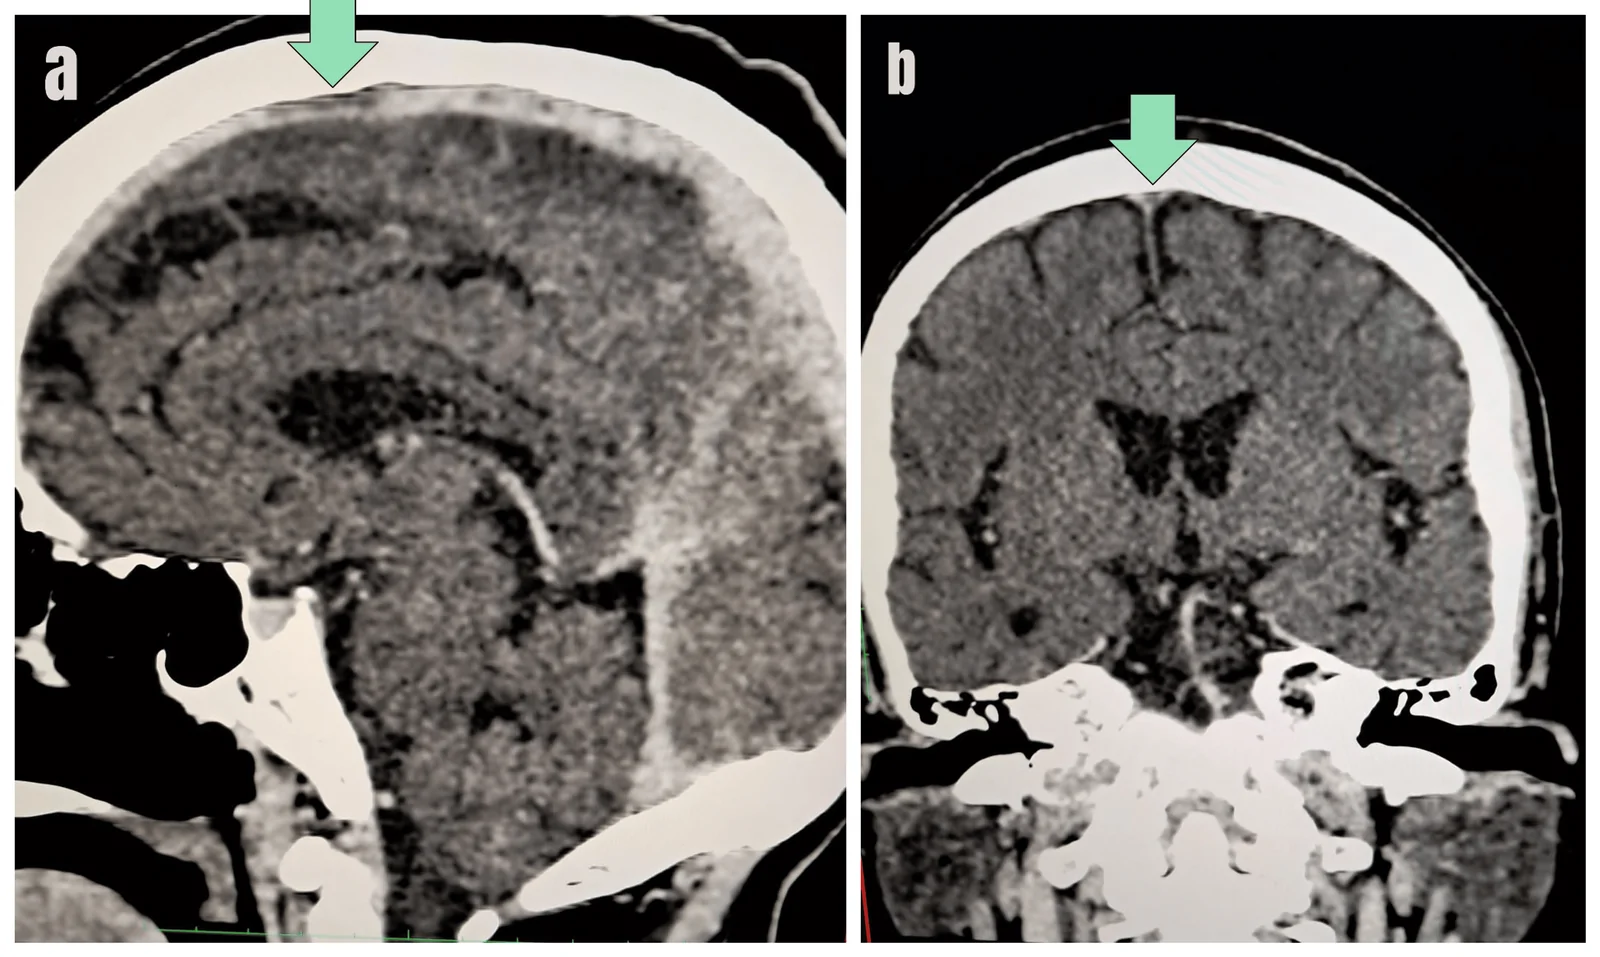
Contrast-enhanced CT scan of the brain (a) Sagittal and (b) coronal views reveal a well-defined intraluminal filling defect within the superior sagittal sinus (indicated by green arrows), representing a non-enhancing clot consistent with acute dural venous sinus thrombosis.
